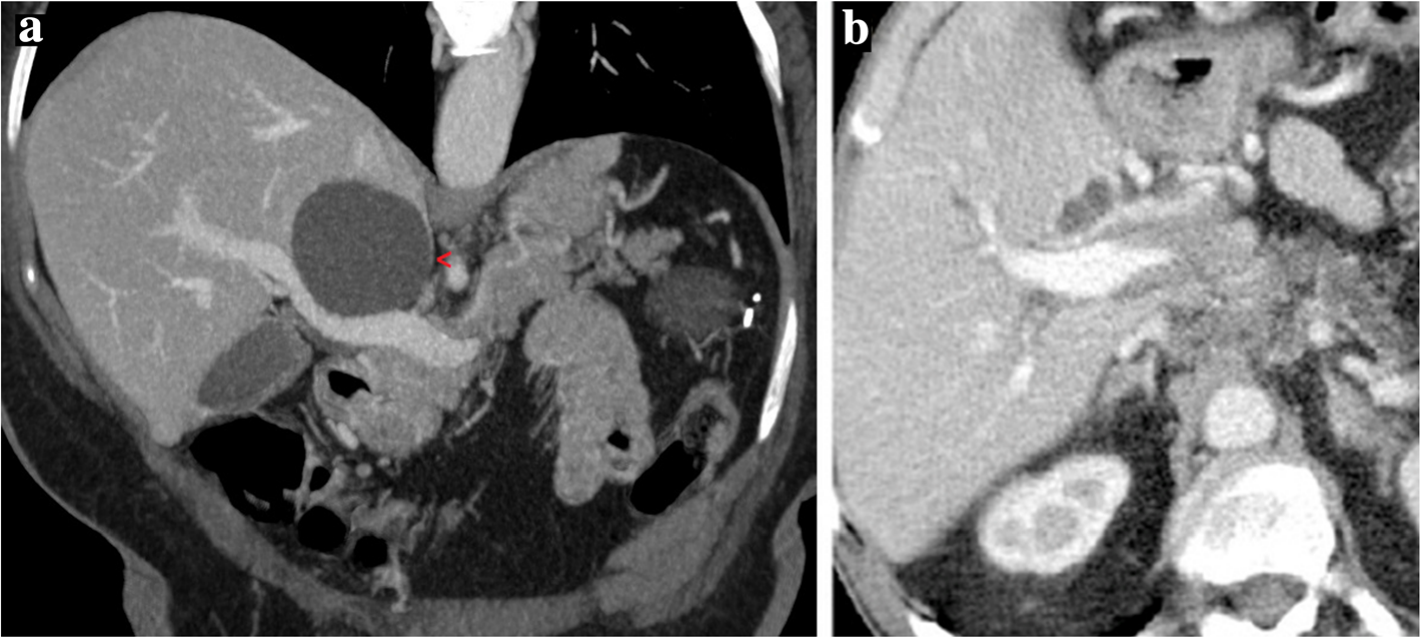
Pre-sinusoidal causes of PH. a MIP image from portal venous phase CT shows extrinsic compression of PV by a large hepatic cyst (red arrow head), a benign cause of pre-sinusoidal PH. b MPR image from portal venous phase CT show PV invasion by a pancreatic adenocarcinoma, a malignant cause of pre-sinusoidal PH
